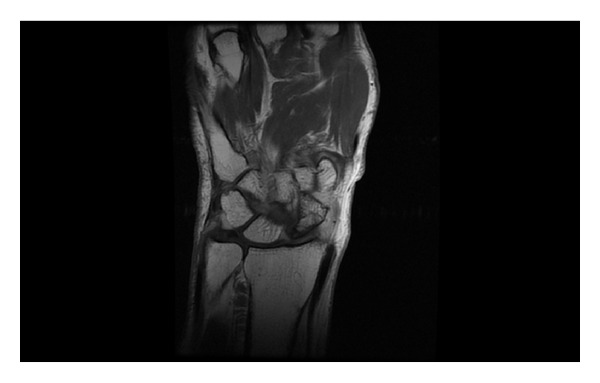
Coronal slice of MRI showing carpus.
